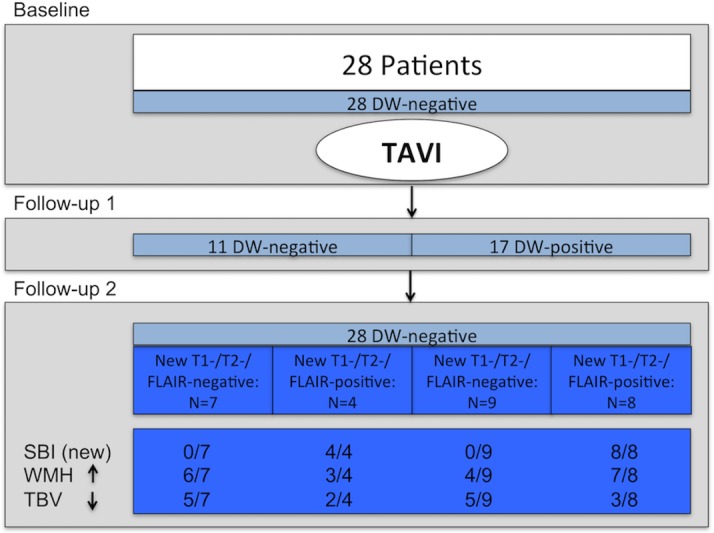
Morphological cerebral outcome after TAVI. The majority of patients (60.7%) had acute lesions in cerebral diffusion-weighted magnetic resonance imaging (DW-MRI) after TAVI. However, there was no association of these acute events (DW-CVEs) and the incidence of SBIs after long-term follow-up. Moreover, DW-CVEs neither aggravated the progression of white matter hyperintensities (> 1.5% / year), nor the progression of cerebral atrophy (> 1% / year). TAVI–Transcatheter aortic valve implantation, DW–diffusion-weighted, CVE–cerebrovascular event, MRI–magnetic resonance imaging, WMH–white matter hyperintensities, TBV–total brain volume, FLAIR—Fluid Attenuated Inversion Recovery.
